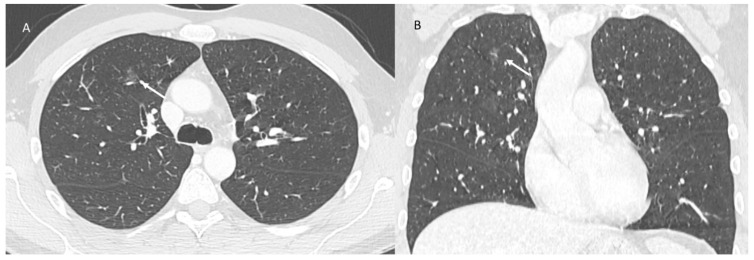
ICI-related pneumonitis. OP pattern on CT (axial: (A) and coronal: (B)): ground-glass and airspace opacities (arrows).
